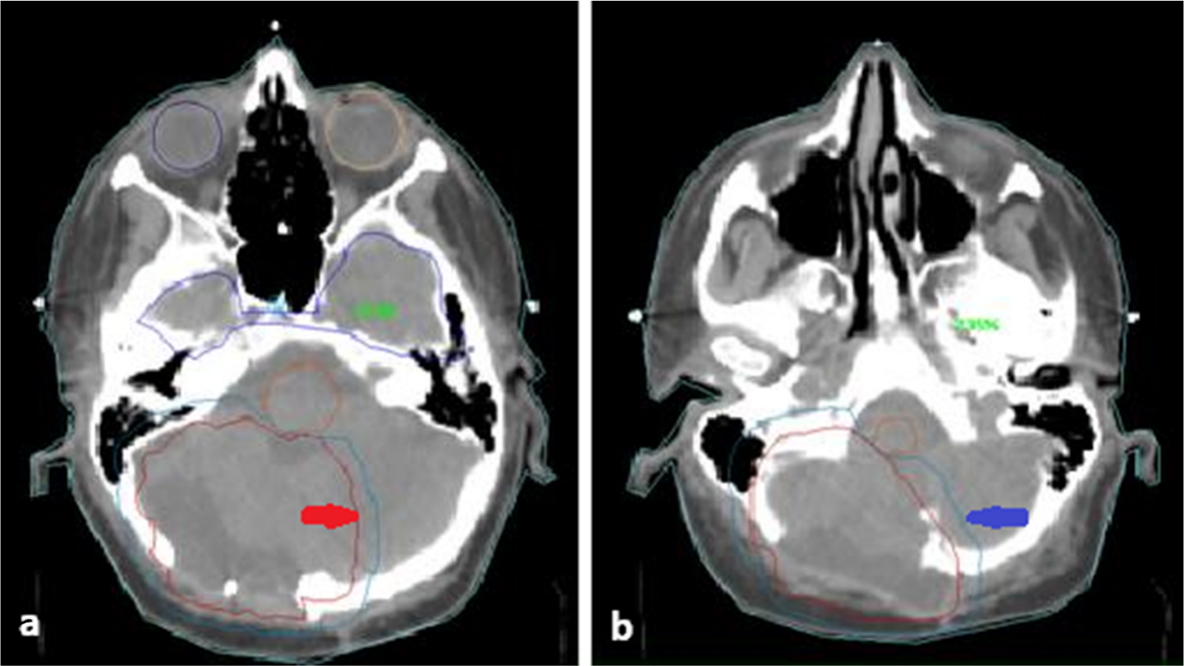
a and b: Planning computed tomography with the retreatment gross tumor volume/clinical target volume and planning target volume identified in red (*red arrow*) and in blue (*blue arrow*), respectively.
